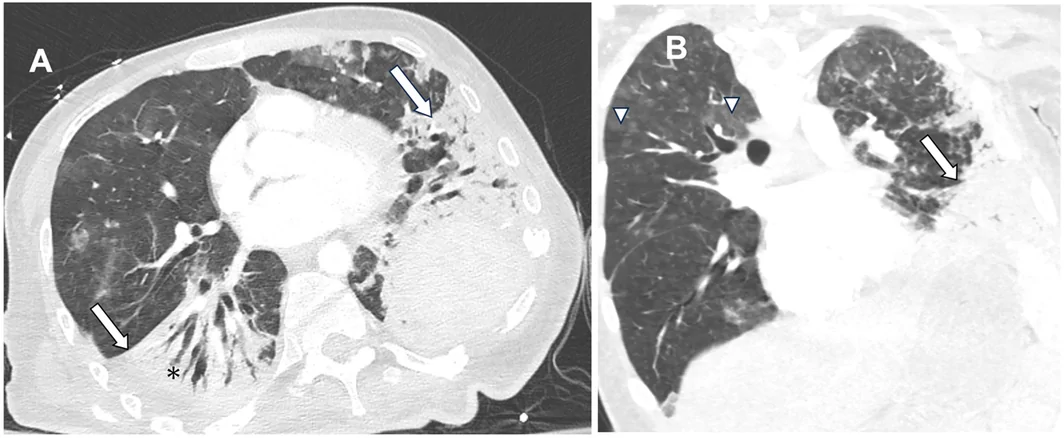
CT images obtained after 21 days of tigecycline therapy. (A) Axial view showing bilateral pulmonary consolidations with marked left‐sided predominance (white arrows), air bronchograms (*), small bilateral pleural effusions and mediastinal lymphadenopathy. (B) Coronal view demonstrating dense left‐predominant consolidation (white arrow), patchy ground‐glass opacities (white arrowheads) and interlobular septal thickening.
